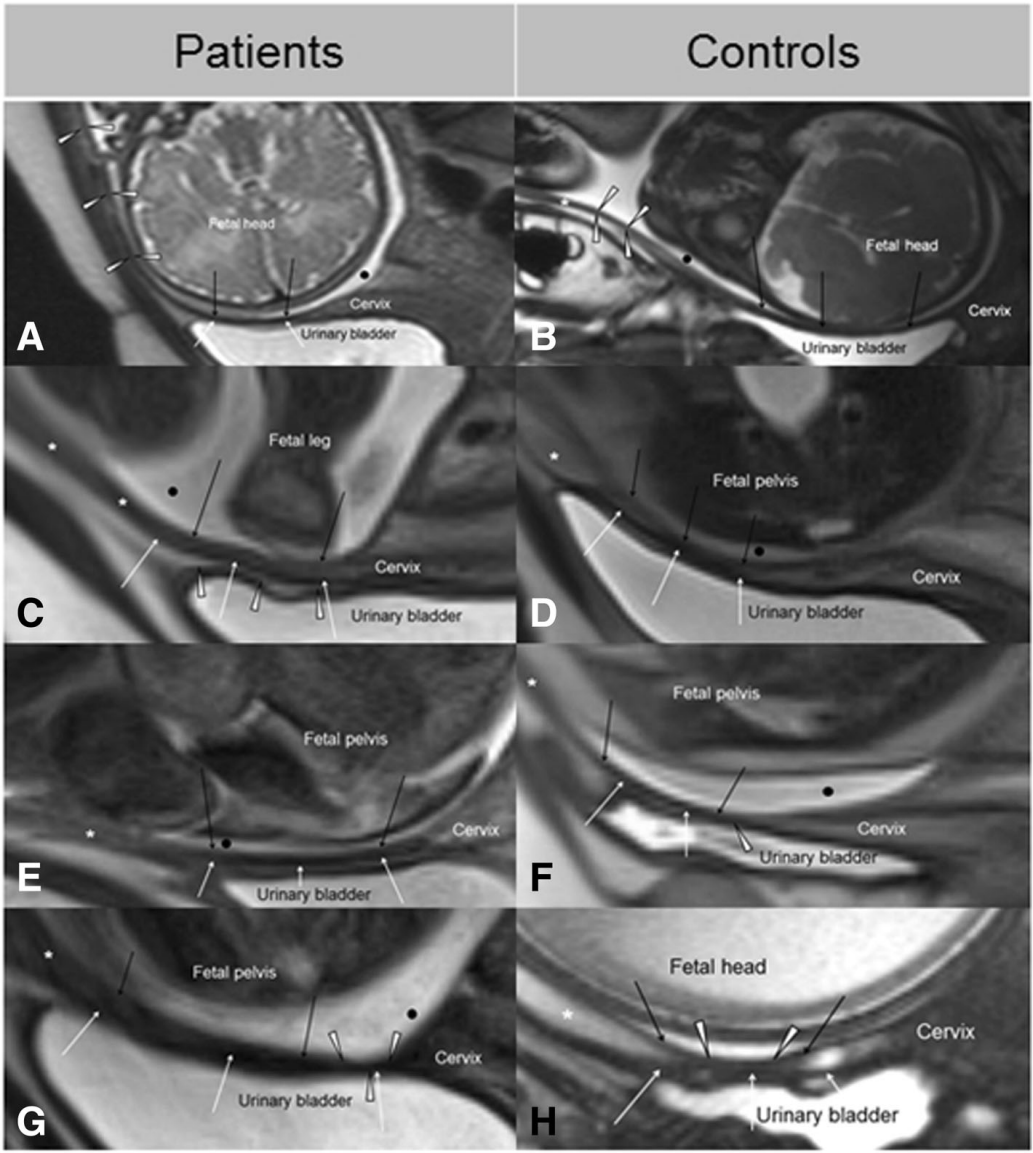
Findings in patients with cesarean section (CS) on the left and in controls without CS on the right. By comparing the images, difficulties in LUS diagnosis and in finding valuable reference values for LUS thickness become clearer. Black point = amniotic fluid, black arrows = inner border of the LUS, white arrows = outer border of the LUS, asterisk = lower uterine front wall, black point = amniotic fluid. As demonstrated in comparison (a) versus (b), thinning of the LUS (thin arrows) and/or the lower uterine front wall (white arrow heads), as well as unclear definitions of LUS and bladder wall occurs in both scarred and unscarred uteri. In contrast, comparison of (c) versus (d) shows that a clear definition of a well-developed LUS from the urinary bladder wall is also commonly found after CS (white arrow heads). Comparison of (e) versus (f) shows that the MRI-signal of the LUS can be hypointense in women with and without previous CS. Even local thinning with an abrupt change of LUS diameter, which is assumed to be the most specific characteristic after CS (g), can probably related to myometrial contractions, occasionally also be observed in women without a uterine scar (f) and (h), (white arrow heads)
